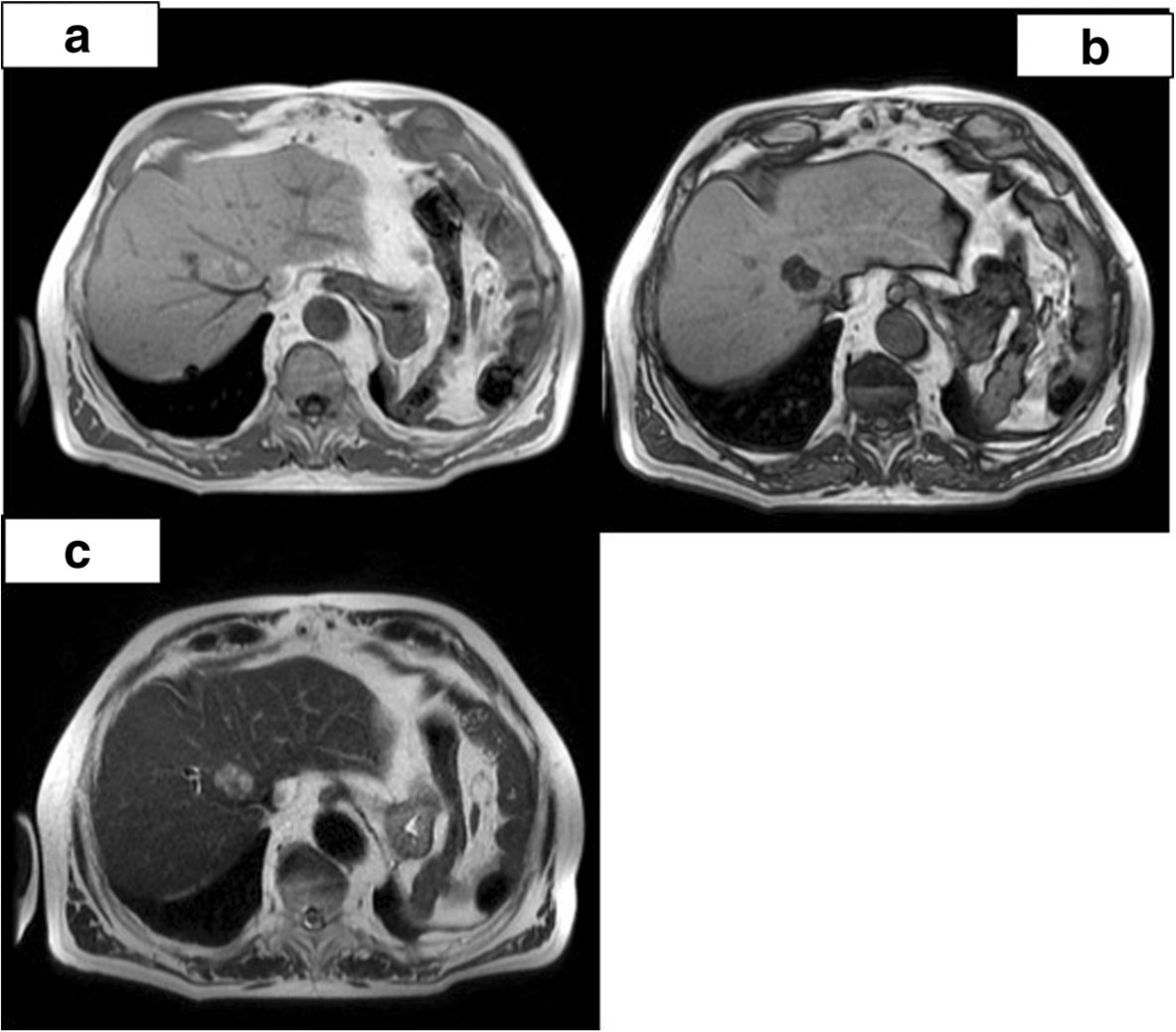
a–c Abdominal MRI (December 5, 2011) confirming the presence of fatty liver lesion; on a–b is shown a signal drop on T1w in phase and opposed phase, respectively, suspected for steatotic adenoma. Lesion appears with internediate signal intensity on T2w image (c)
